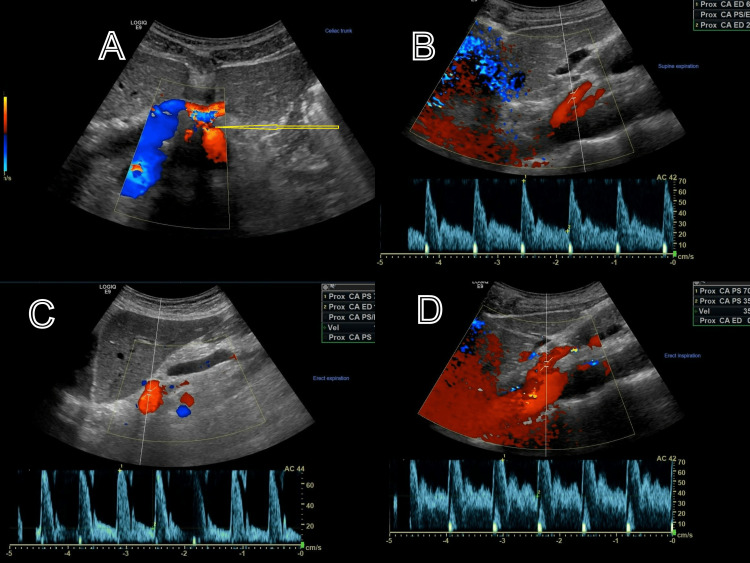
Doppler Ultrasound with the arrow showing the point at the constriction of the different variations of flow through the celiac trunk. A: constricted portion of the celiac artery. B: supine expiration. C: erect expiration. D: erect inspiration.
